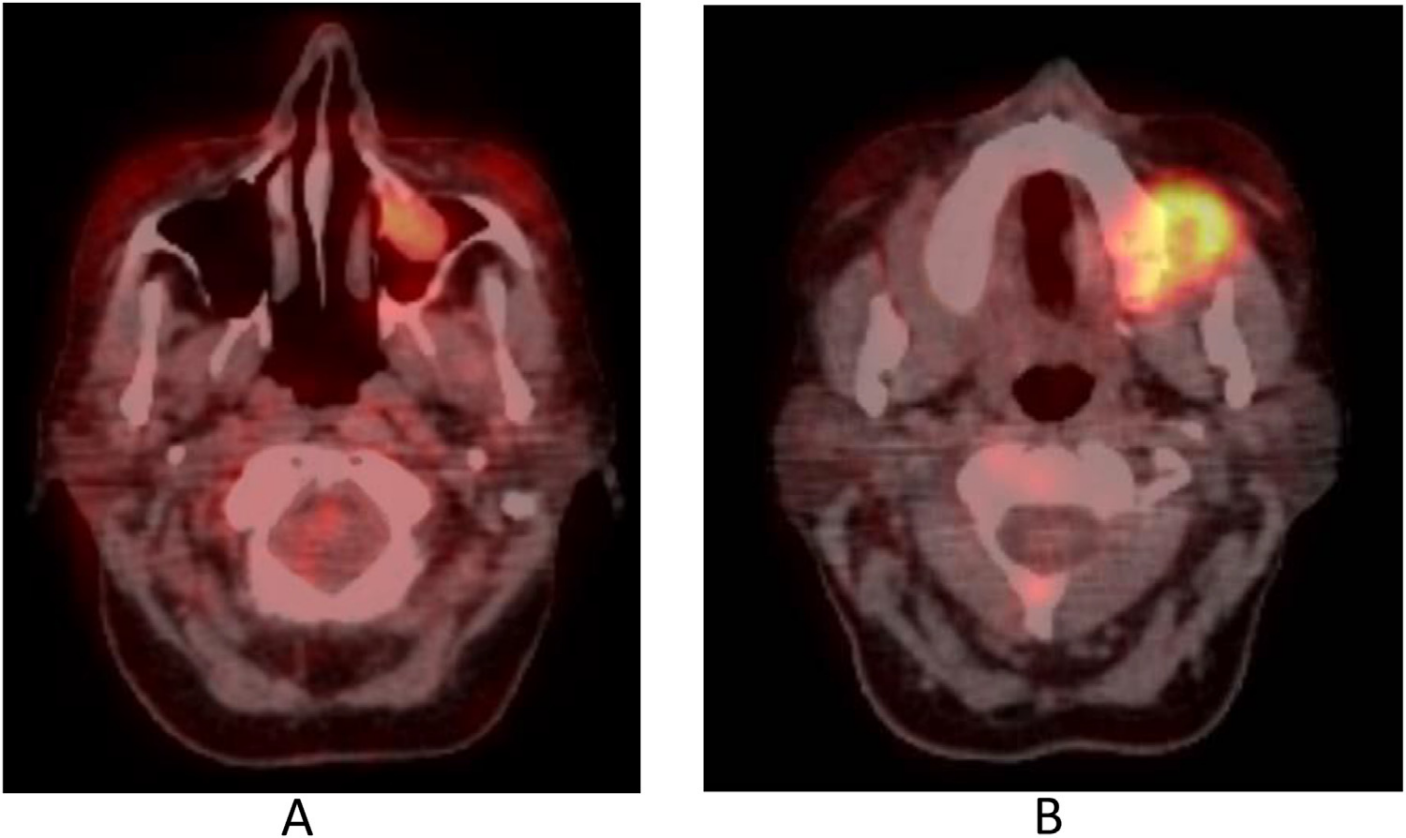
(A, B) PET/CT confirmed the presence of an aggressive lesion showing an increased metabolic activity in the left maxillary sinus (SUV from 5.6 to 15.3).
